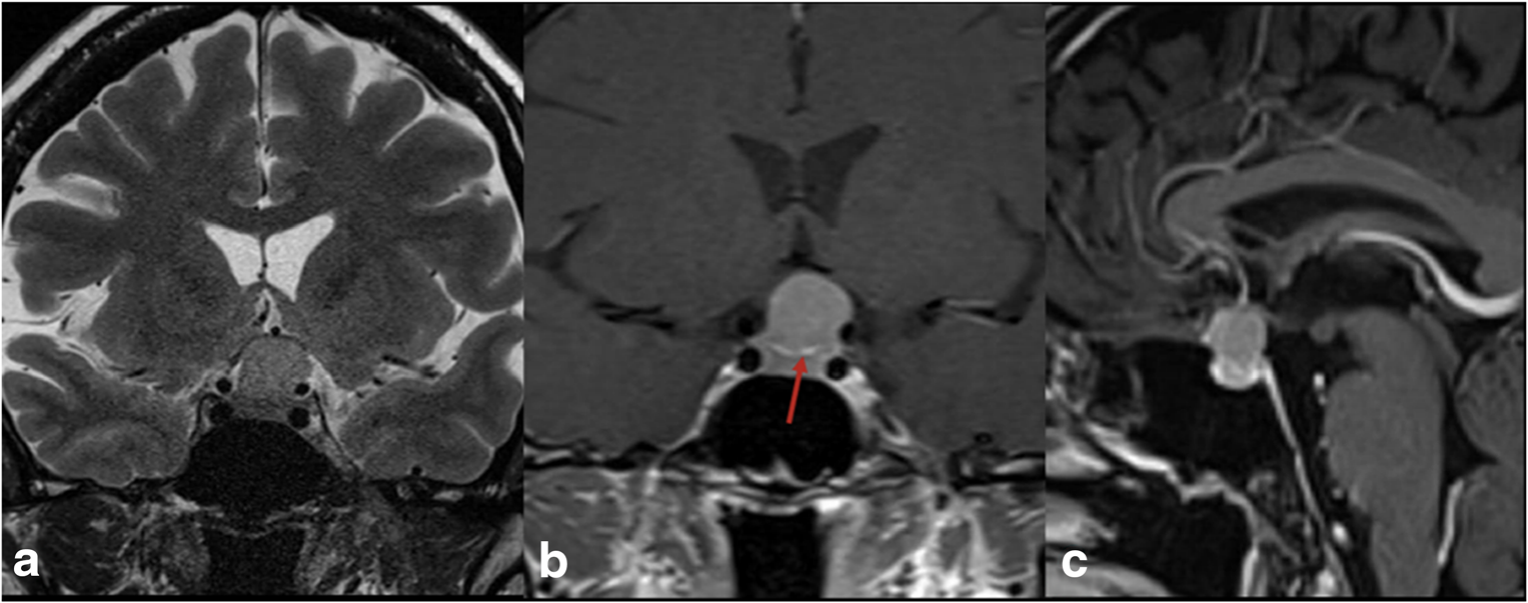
Preoperative MRI of a 45-year-old lady who presents with progressive decrease in visual acuity of the left eye. a T2W coronal MR image showing a homogenous tumor involving the suprasellar region extending laterally to the left internal carotid and superiorly to the chiasm which is compressed by the lesion. b Gd-enhanced T1 W coronal MR image showing the suprasellar lesion with enhancement of the diaphragma sellae (red arrow) separating the lesion from sellar contents. c Gd enhanced T1W sagittal MR image showing the widest attachment base of the tumor localized at the diaphragma sellae
